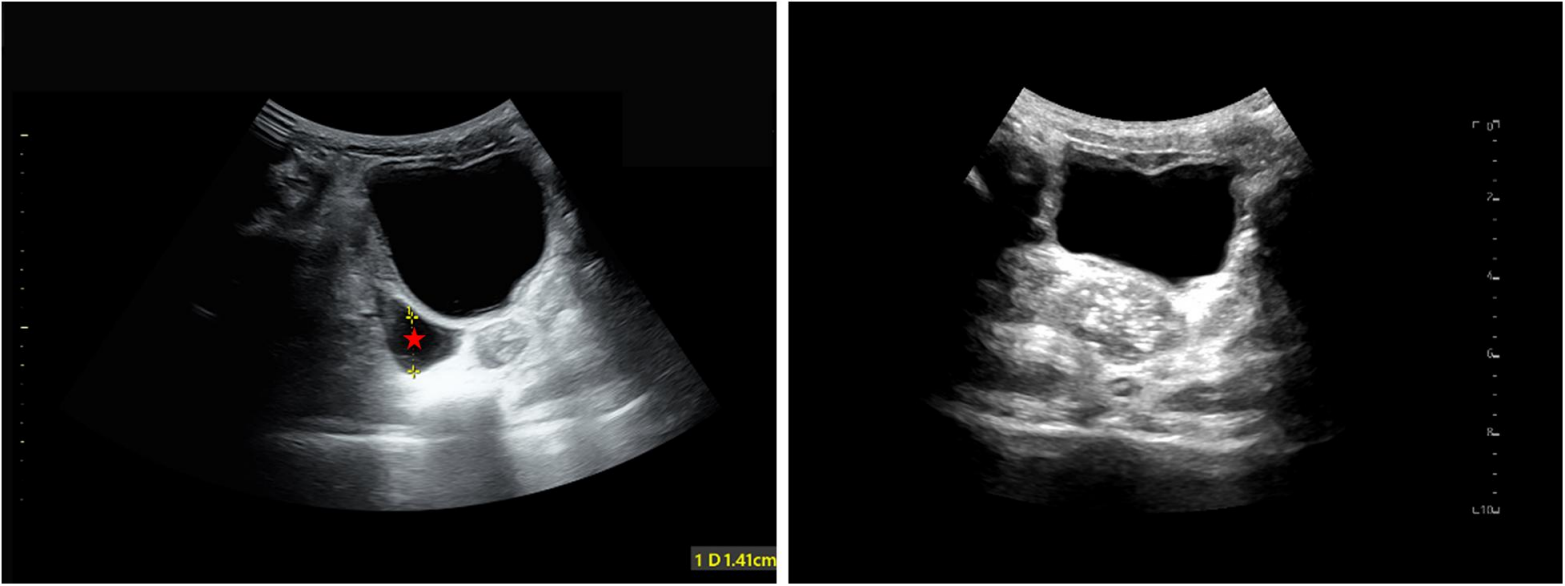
Doppler ultrasonography revealed a small amount of fluid in the lower abdomen with a maximum depth of 1.4 cm (left, red star), which was no longer present after treatment (right).
